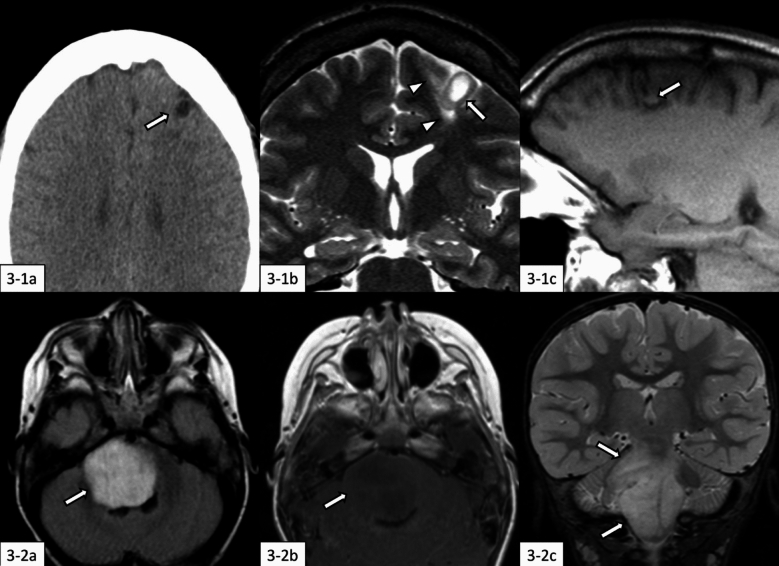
Angiocentric glioma. 3-1 (43-year-old man): There is a tumor mass with the cortical and subcortical involvement in the right frontal lobe (3-1a–c, arrows). The tumor mass shows hypodensity on non-contrast computed tomography (3-1a), hyperintensity on T2-weighted coronal imaging (3-1b), hyperintense rim and central hypointensity on T1-weighted sagittal imaging (3-1c). The hyperintense “stalk-like sign” in the surrounding brain parenchyma toward the lateral ventricle is observed on T2-weighted coronal image (3-1b, arrowheads). 3-2 (2-year-old boy): There is a tumor mass in the pontomedullary region (3-2a–c, arrows). The tumor mass shows hyperintensity on fluid-attenuated inversion recovery (3-2a) and hypointensity without contrast enhancement on contrast-enhanced T1-weighted imaging (3-2b). Fat-saturated T2-weighted coronal imaging shows infiltrating growth of the tumor mass with an ill-defined margin (3-2c), mimicking diffuse midline glioma, H3K27-altered. The same cases with different images have been evaluated in our previous study [22]
